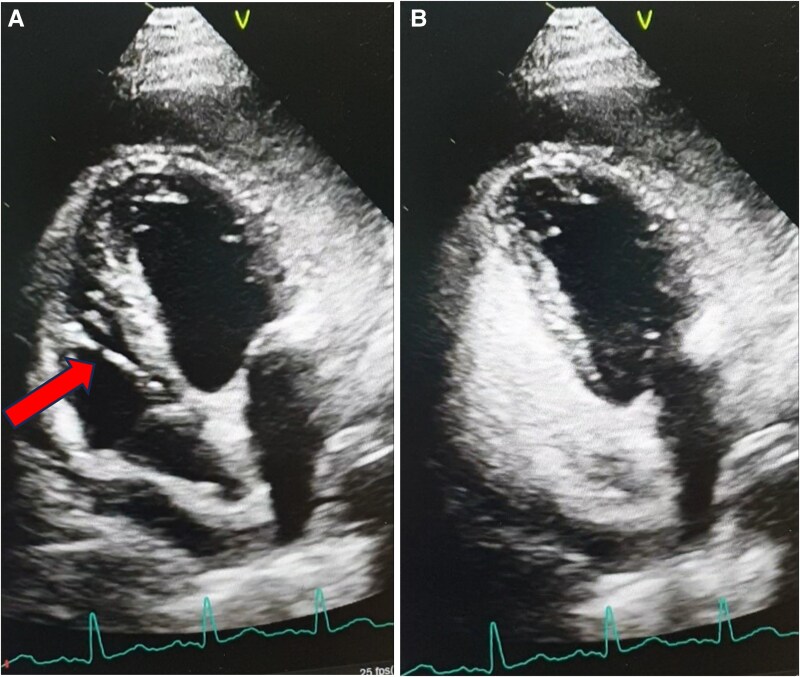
(*A*) Right ventricle perforation evidenced by the presence of pigtail catheter which was seen by echocardiography inside right ventricle. (*B*) Echocardiography image shows that agitated saline is seen inside the right-side cavity of the heart.
